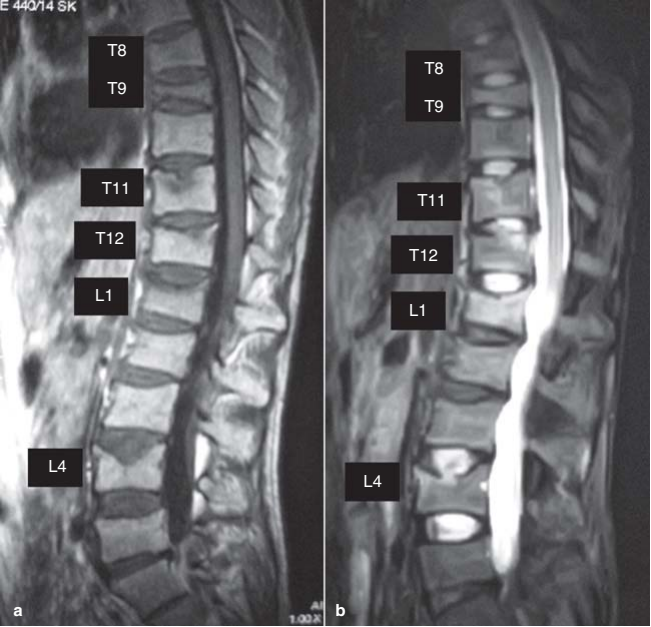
Magnetic resonance imaging (MRI) of the vertebrae in case 2. MRI showed low-signal intensities on T1-weighted images (A) and high intensities on T2-weighted images (B) at the 8th, 9th, 11th, and 12th thoracic vertebrae and 1st and 4th lumbar vertebrae.
